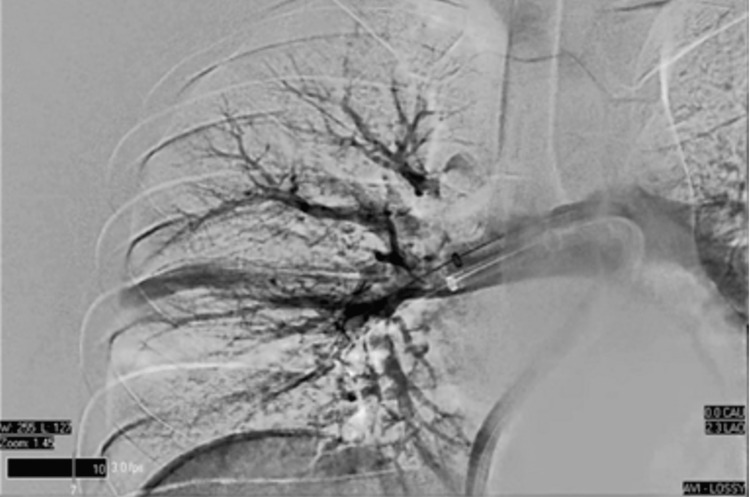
Ultrasound-guided right femoral vein access catheterization of the right lung
